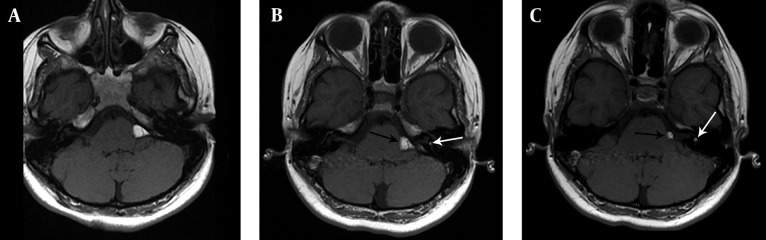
A, B and C) T1-weighted MRI images show lipoma presenting as hyperintense lesions in the left cerebellopontine angle (black arrows) and the left internal acoustic canal (white arrows).
